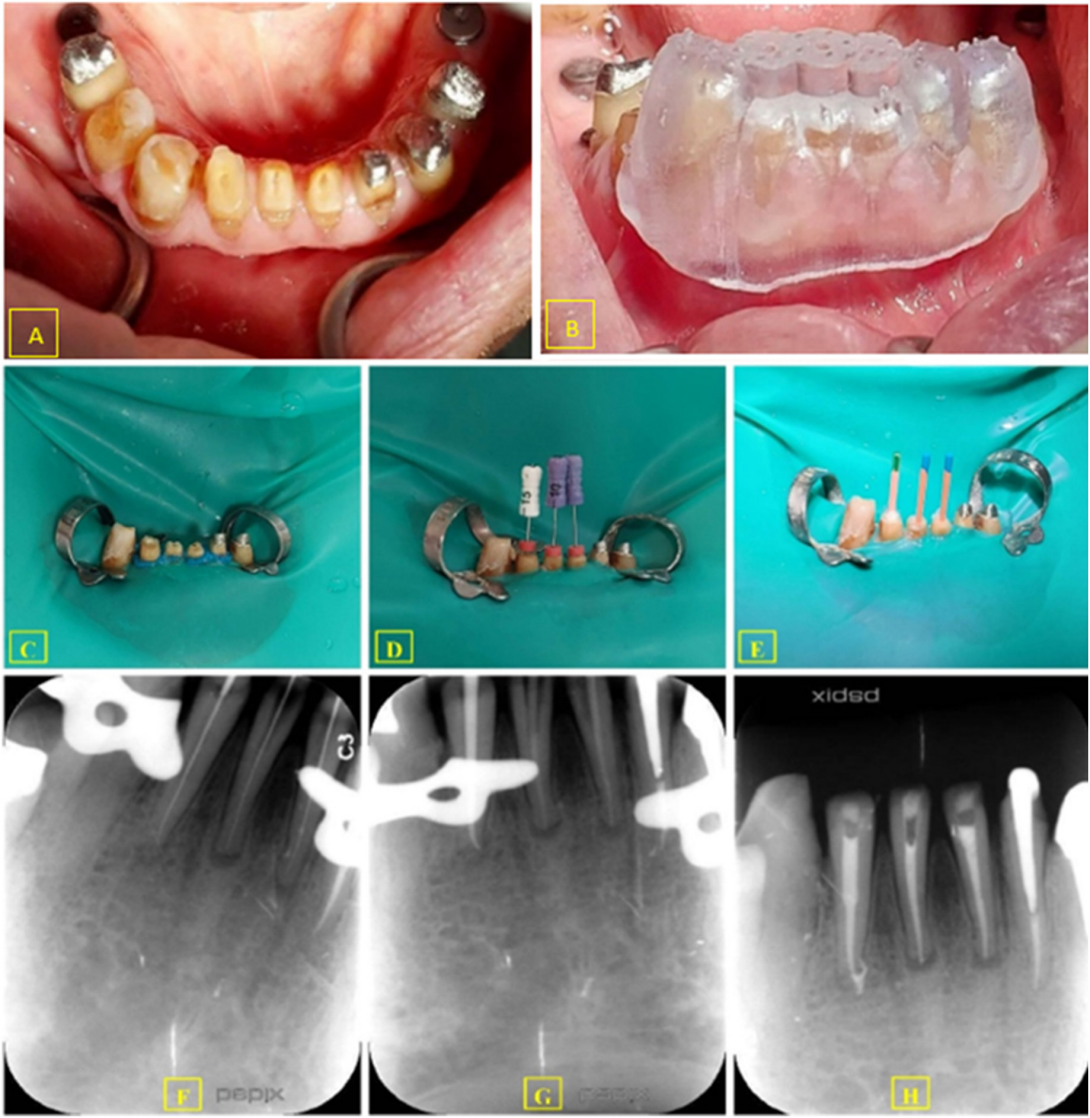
The clinical procedure. (A) The teeth after incisal reduction; (B) checking the correct positioning of the 3D guide; (C) isolation with rubber dam; (D) negotiation of root canals with K‐files; (E, F) determination of the working length; (G) cone fit; (H) post‐obturation radiograph.
